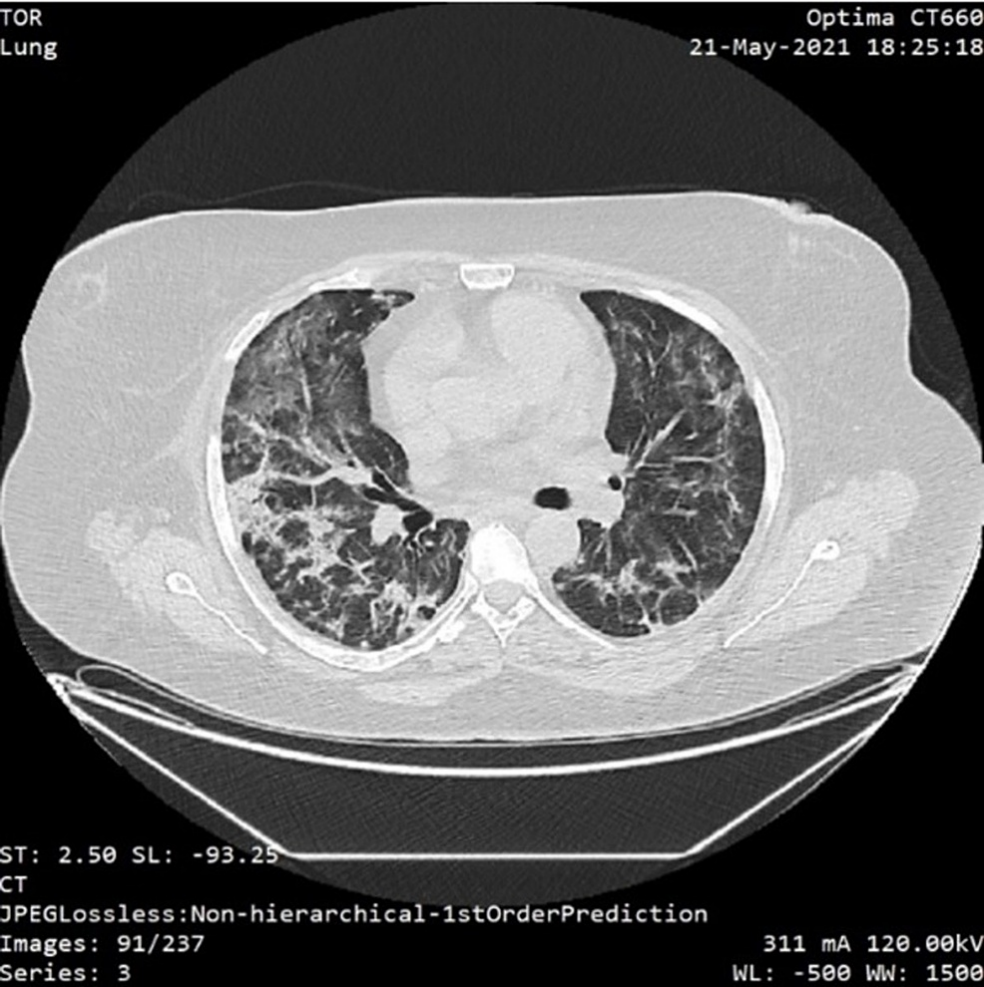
Thorax CT scan showing typical fibrotic lesions associated with bronchiectasis extensive distributed to the right lung and 2/3 of the left lung (over 75% lung involvement)
